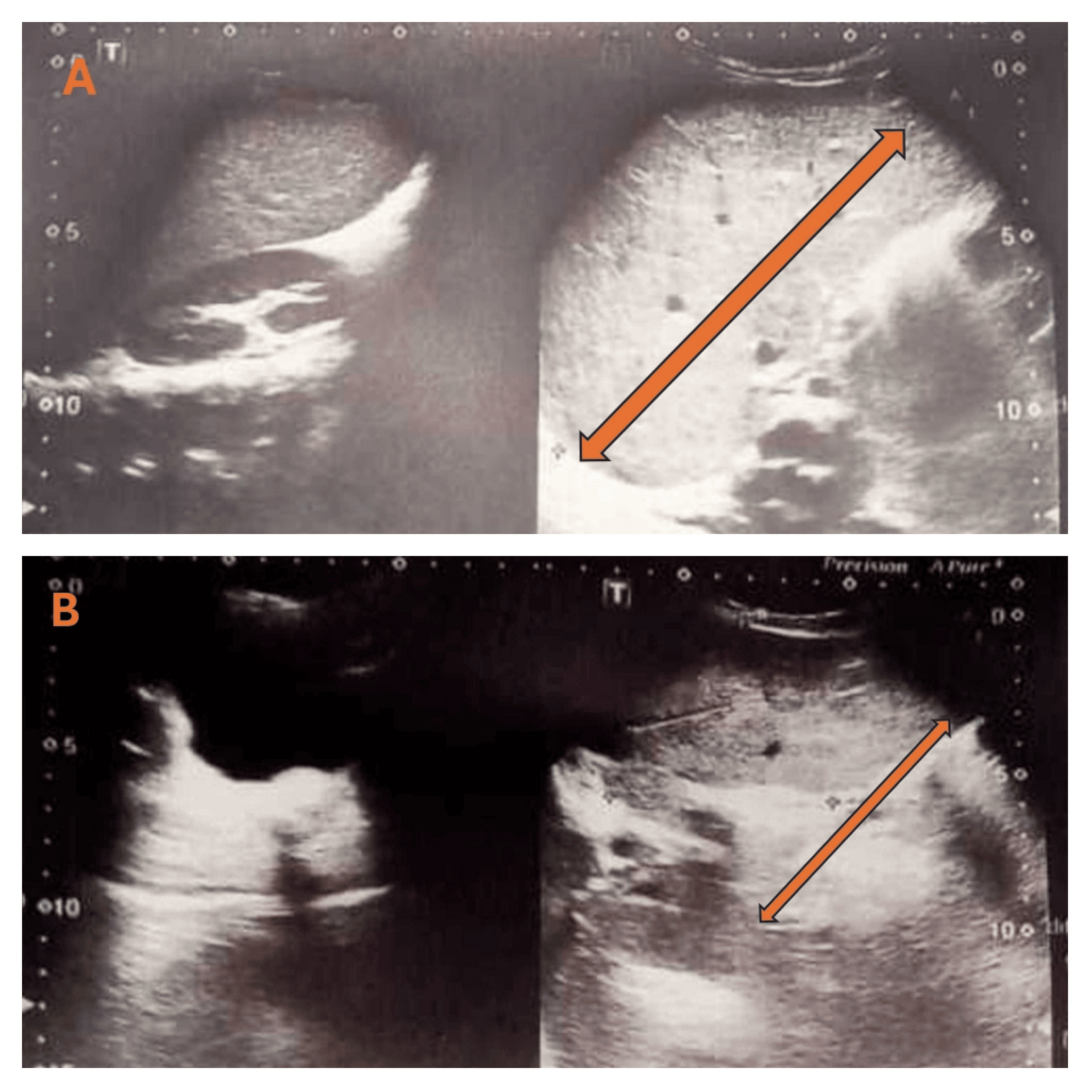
Ultrasound abdomen for organomegaly A: Enlarged liver; the arrow shows the size of the liver. B: Enlarged spleen; the arrow shows the size of the spleen.
